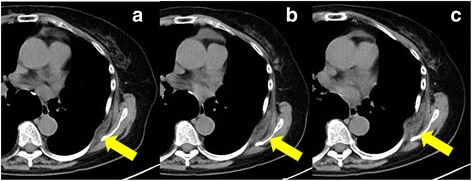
a CT scan at 4 years after surgery shows a subscapular mass with a density similar to that of the skeletal muscle with moderate enhancement. b, c CT scans at 5 and 6 years after surgery shows gradual growth of the subscapular mass over time
